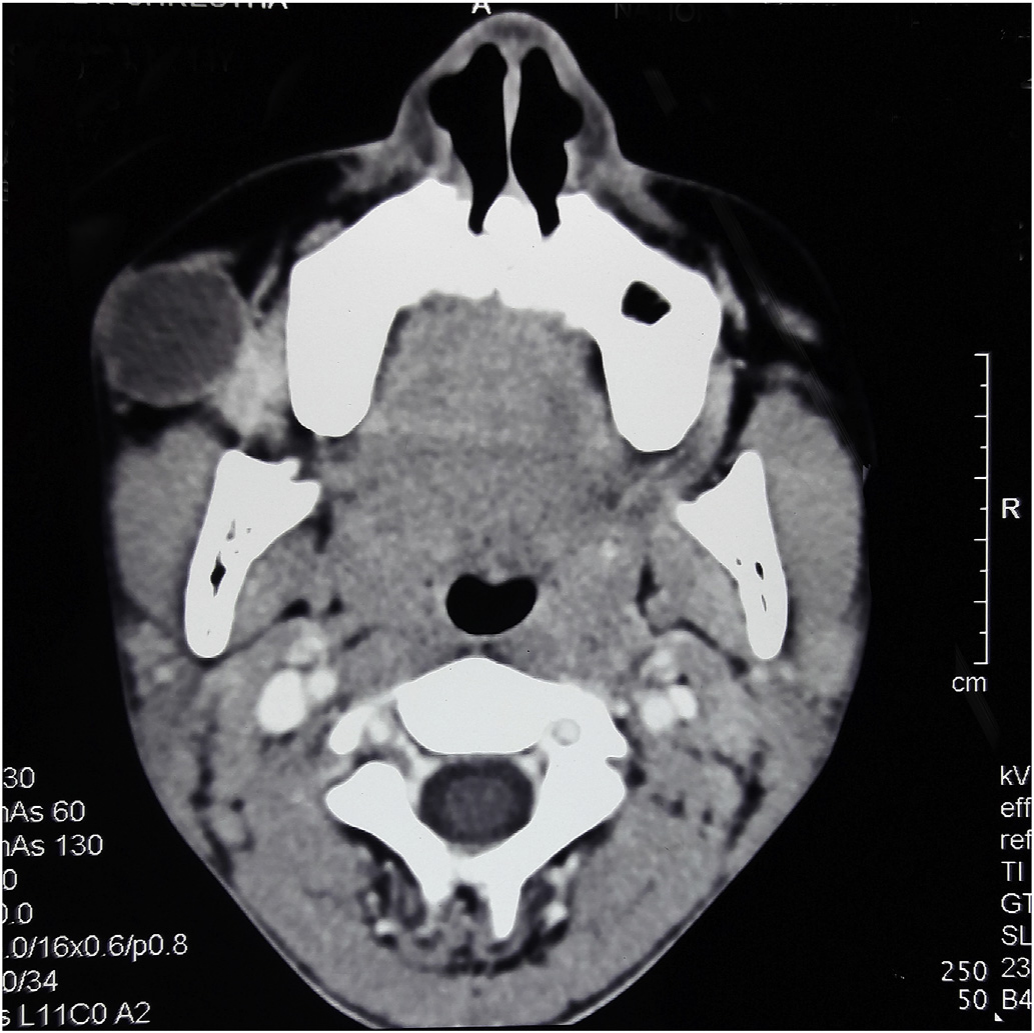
CT scan of head showing cystic lesion in the right buccal space.
